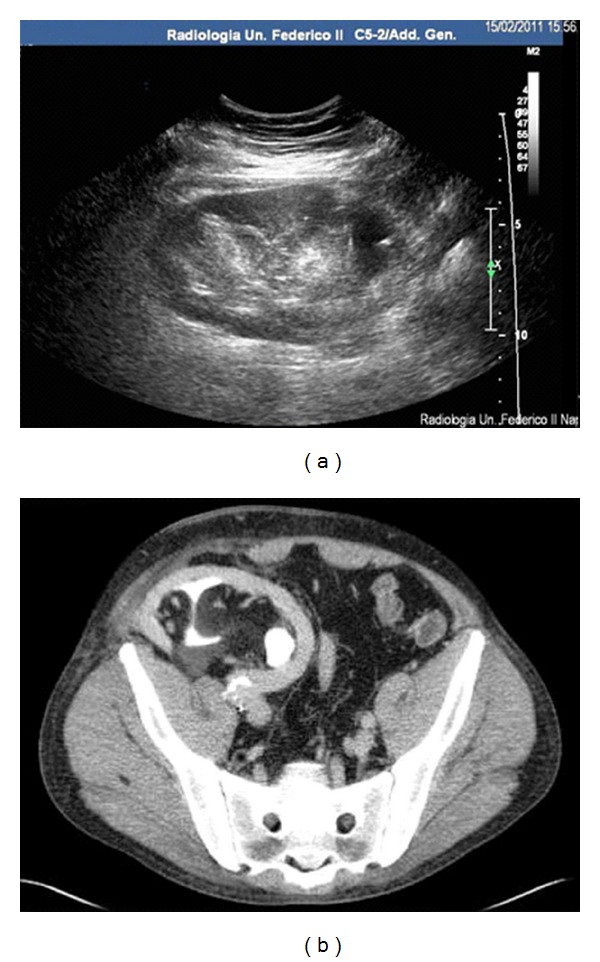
(a) US and (b) CT scan (with contrast medium) performed after the resolution of acute renal impairment, showing the persistence of a mild dilatation of upper calyces and the impregnation with contrast media of pelvicalyceal structures. The entity of perirenal fat is not modified.
